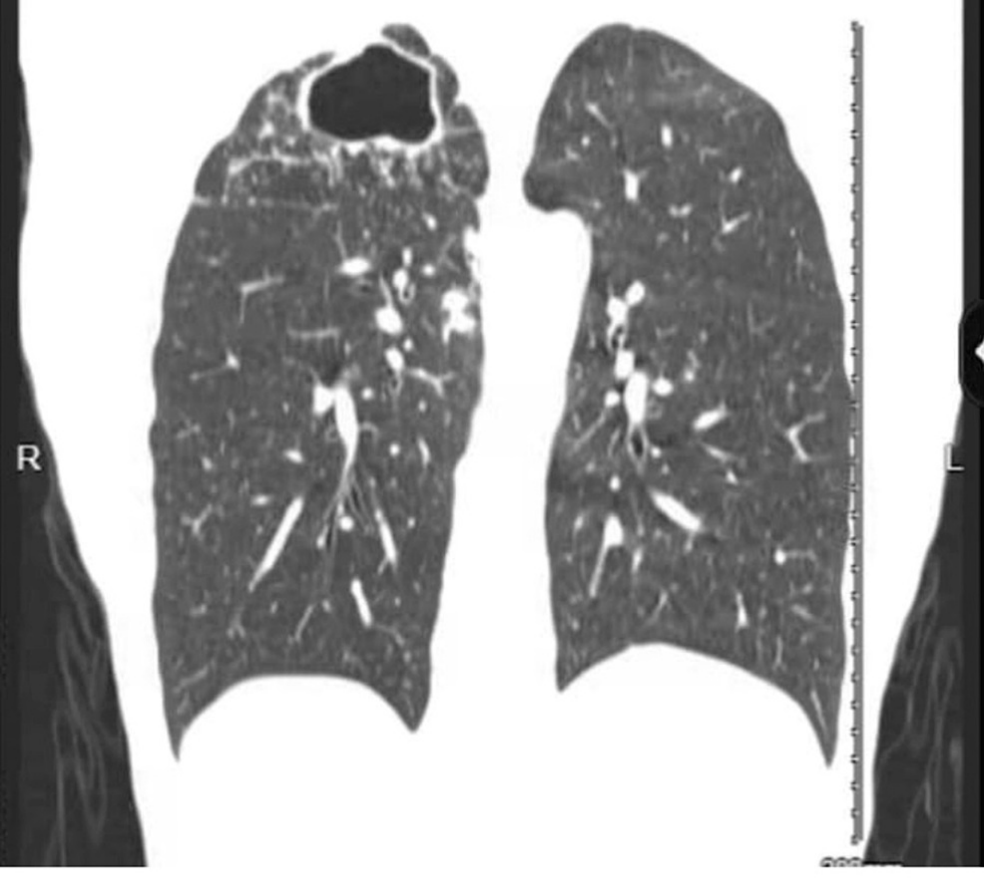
Chest CT revealing a 5.0 × 5.3 cm thick-walled cavitary lobe CT: computed tomography
